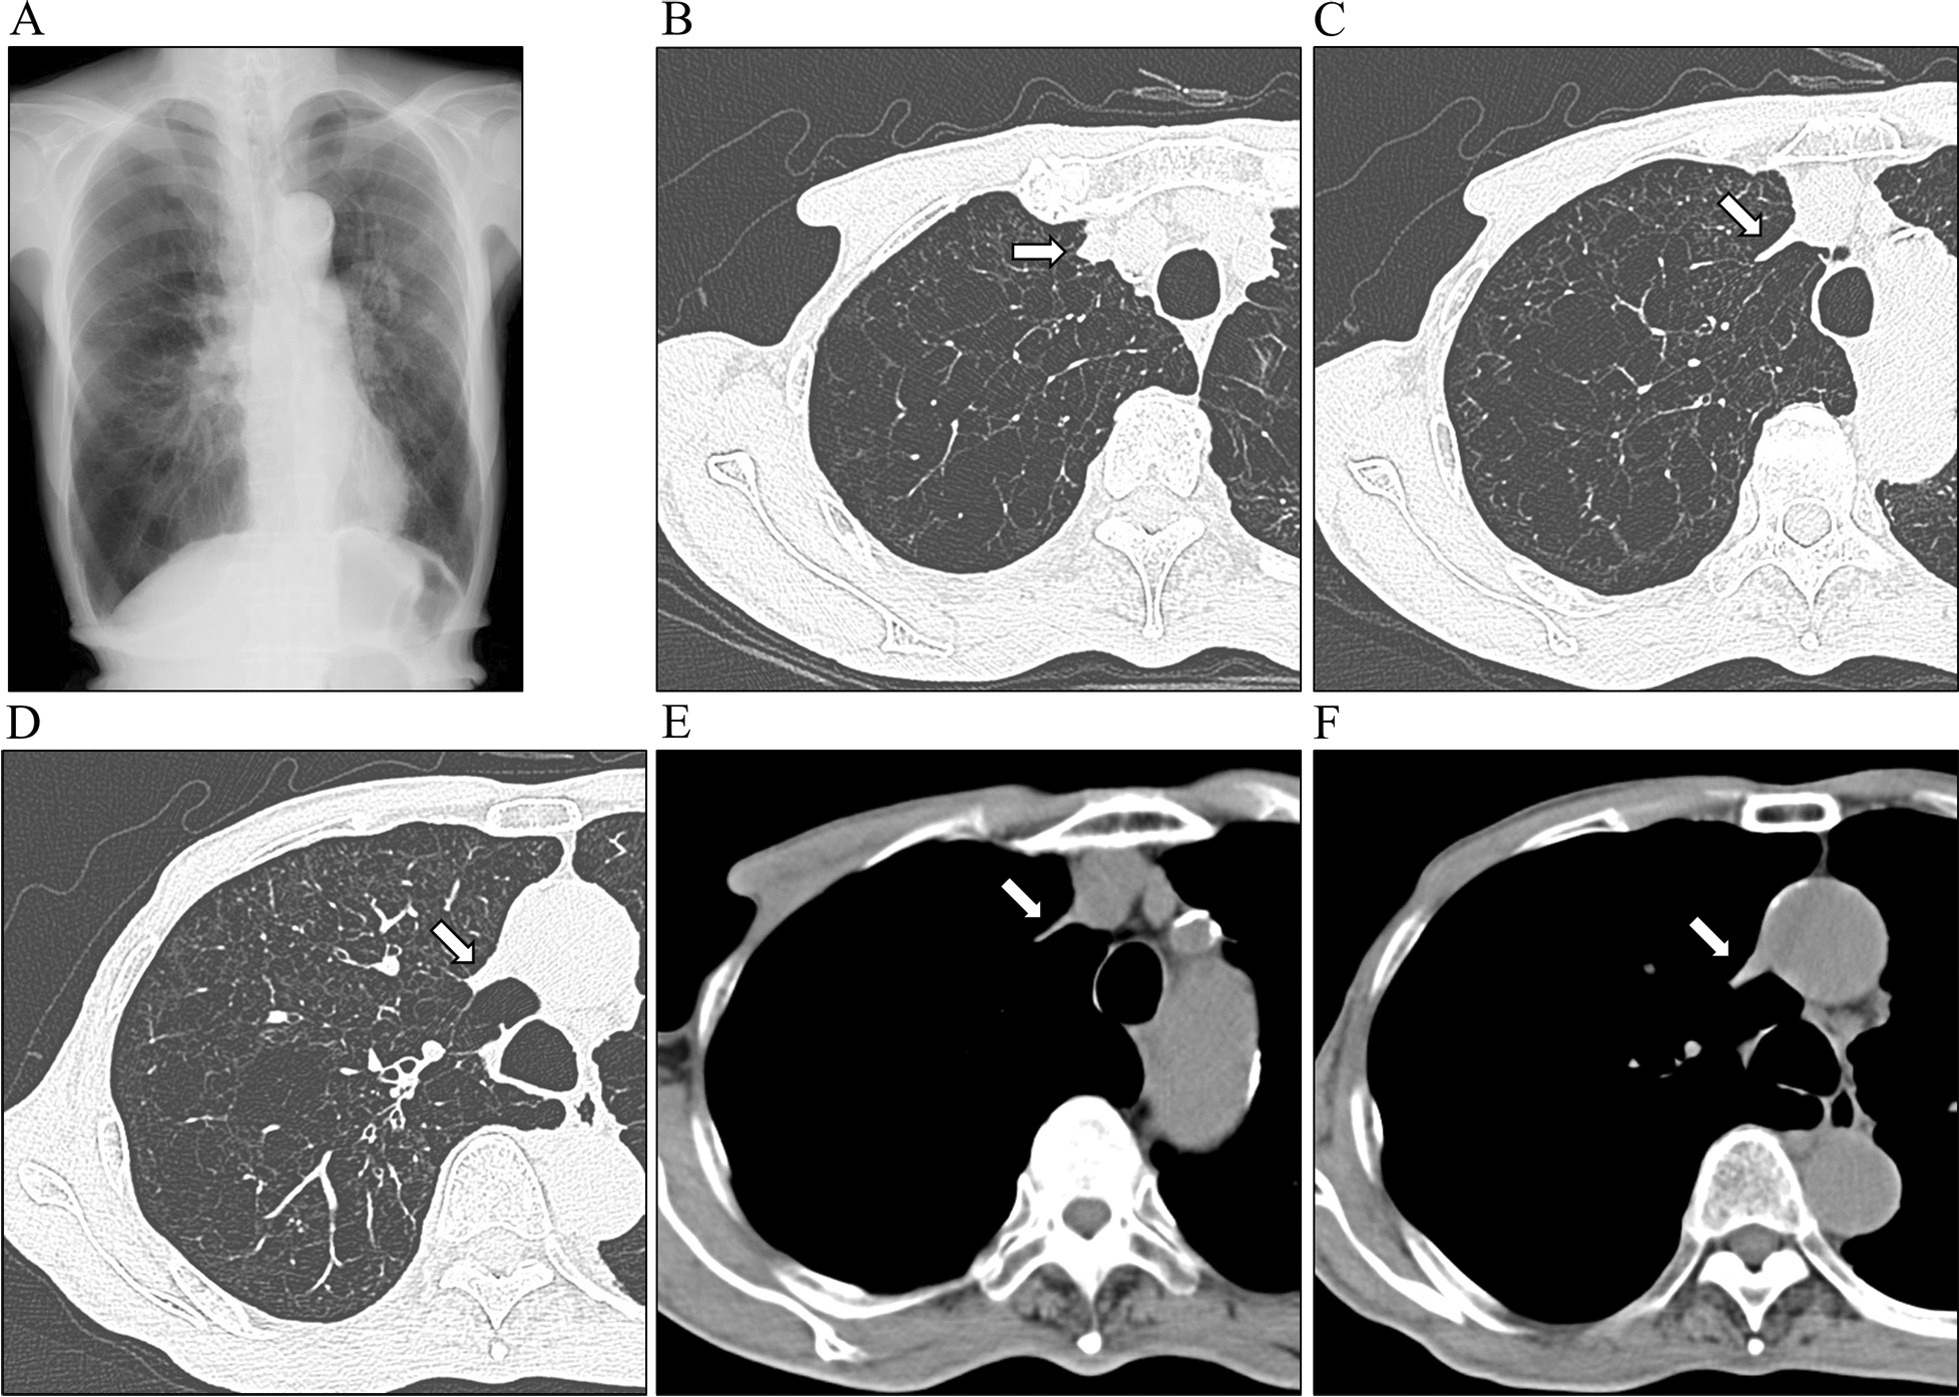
A Chest X-ray showing hyperinflation of the lung field. B–F Chest computed tomography showing emphysematous change. Arrows indicate the superior vena cava, which is partly extremely narrow
